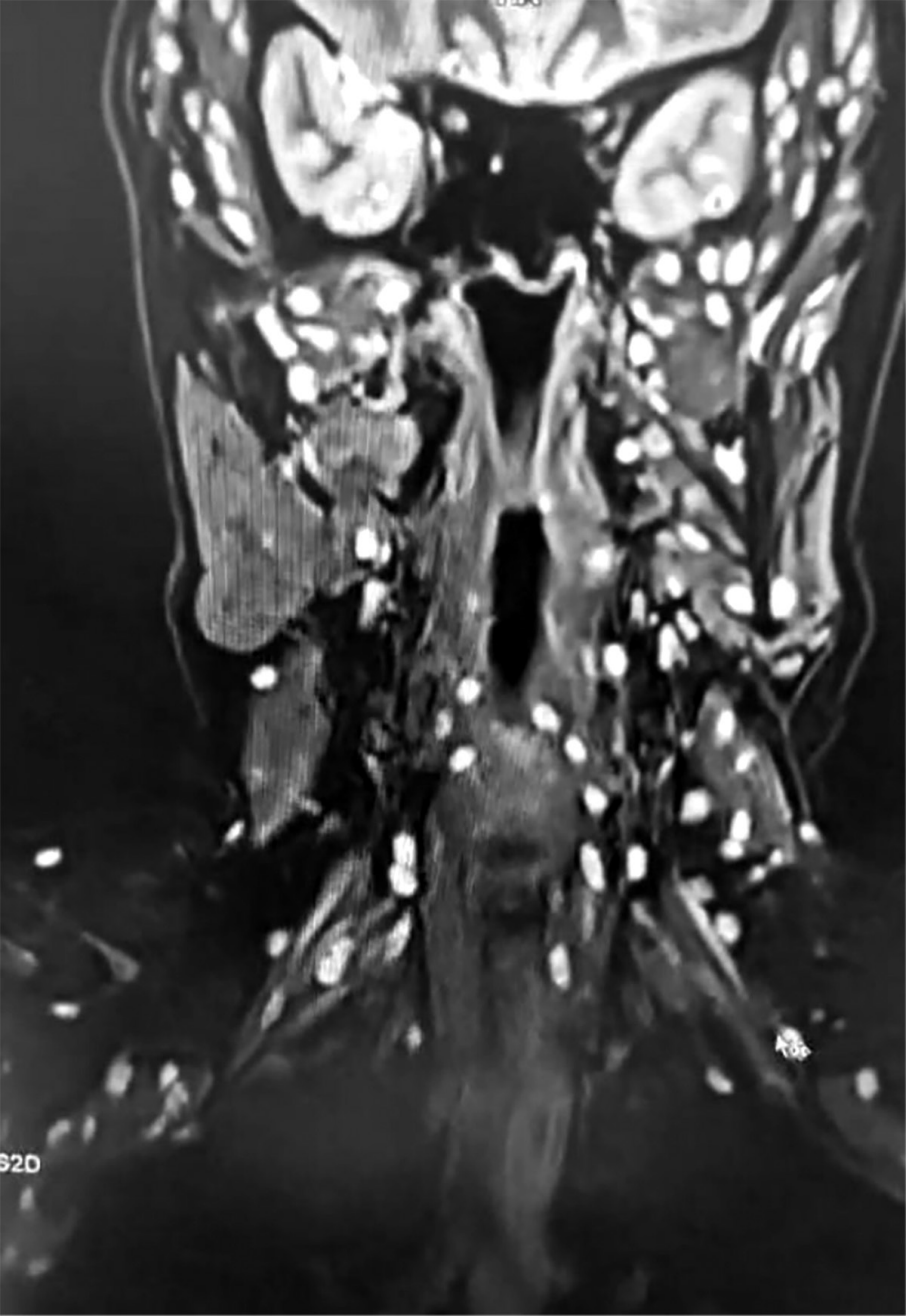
Description: Coronal T2-weighted MRI of the abdomen and retroperitoneum reveals numerous well-defined cystic lesions distributed along the paravertebral and retroperitoneal regions, which is consistent with disseminated cysticercosis. Multiple lesions with eccentric scolex are evident, involving muscle planes and soft tissues. Kidneys are seen symmetrically in the upper field with preserved corticomedullary differentiation. The imaging features are characteristic of systemic cysticercosis with muscular and subcutaneous involvement. Clinical Significance: The presence of multiple cysticerci within retroperitoneal and paravertebral regions confirms disseminated cysticercosis, an uncommon but clinically significant form of the disease. Identification of the scolex within lesions differentiates cysticercosis from other parasitic or neoplastic cystic pathologies. Preservation of renal morphology suggests no secondary renal involvement, underscoring the systemic muscular and subcutaneous distribution pattern. Origin: @GSVM Medical College, PMSSY Superspeciality Hospital, Kanpur, Uttar Pradesh, India. Pincode: 208002.
